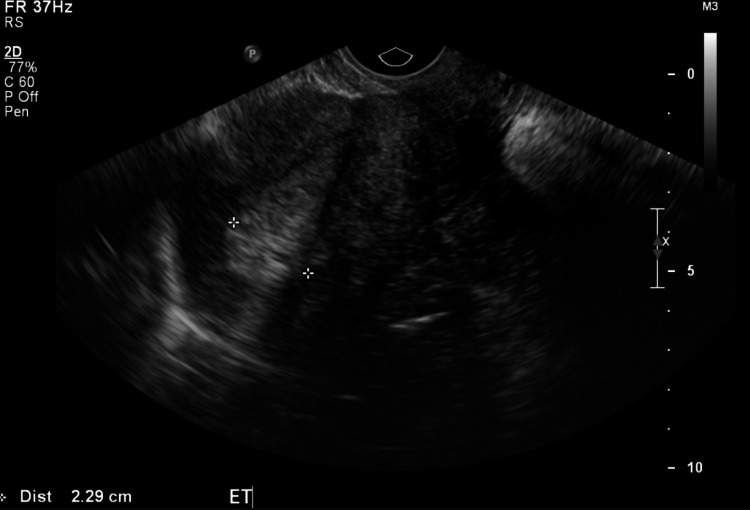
Ultrasound of the uterus showing increased endometrial thickness in the area marked by asterisks.
